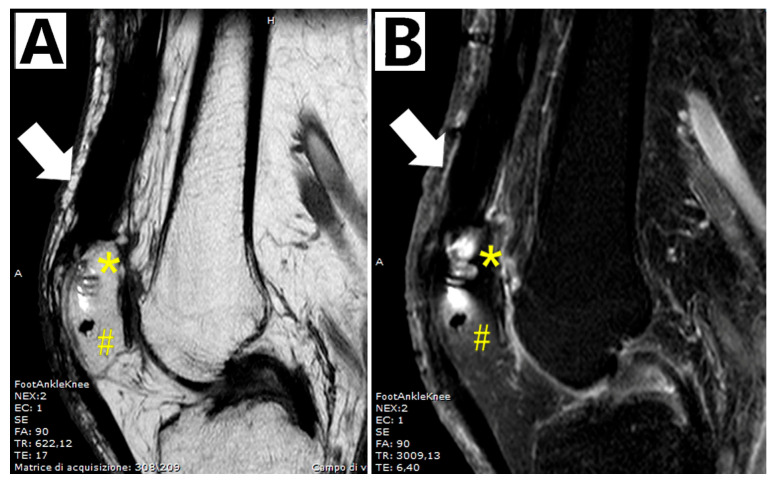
MRI at 5-year follow-up. (A) Spin-echo (SE), T1-weighted; (B) fast spin-echo, fat-saturated, T2-weighted. Continuity of the reconstructed quadriceps tendon with complete graft incorporation: quadriceps tendon (white arrow); suture anchor (*); patella tunnel (#).
